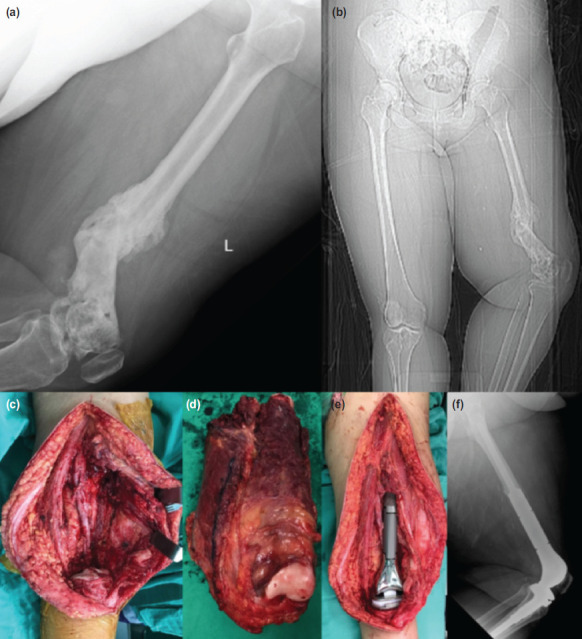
(a,b) Radiograph of left femur deformity with callus formation and angulation of distal fragment anterolaterally in keeping with malunion, following chemotherapy and prolonged antibiotic. (c,d,e) Wide resection margin of bone and surrounding soft tissue to achieve good local control for megaprosthesis reconstruction. (f) Post-operation radiograph of distal left femur shows megaprosthesis in situ.
